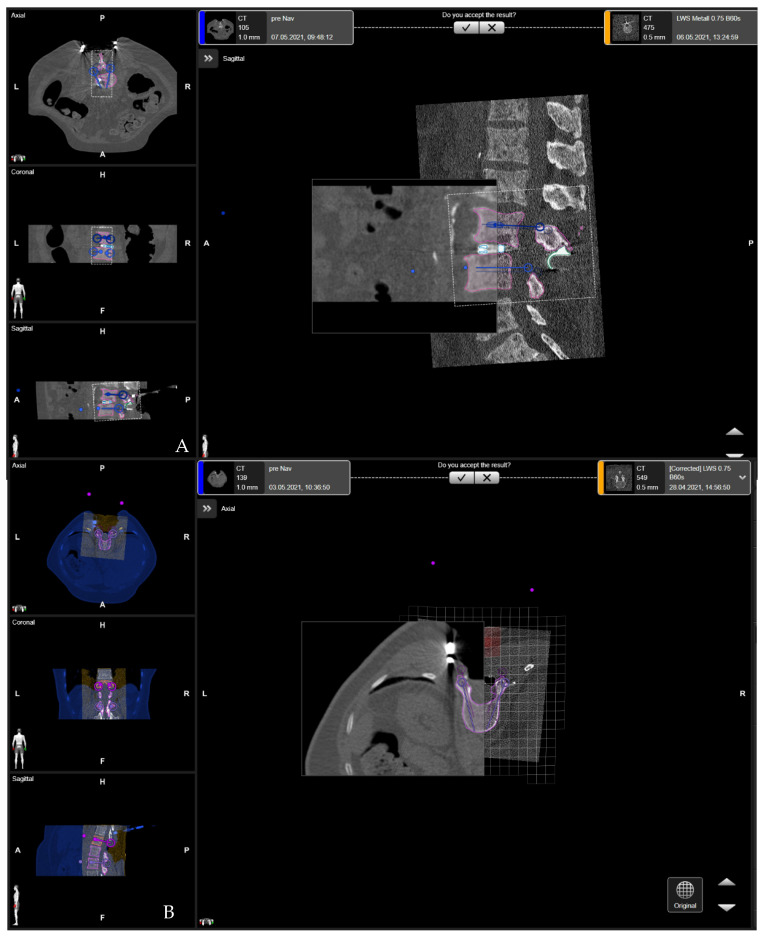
(A). Rigid fusion of the preoperative CT of the lumbar spine and registration iCT scan with preplanned screw trajectories (Patient number 3). (B). Elastic fusion of the preoperative CT of the lumbar spine and control iCT in the case of multisegmented stabilization in the thoracolumbar spine (Patient number 2).
